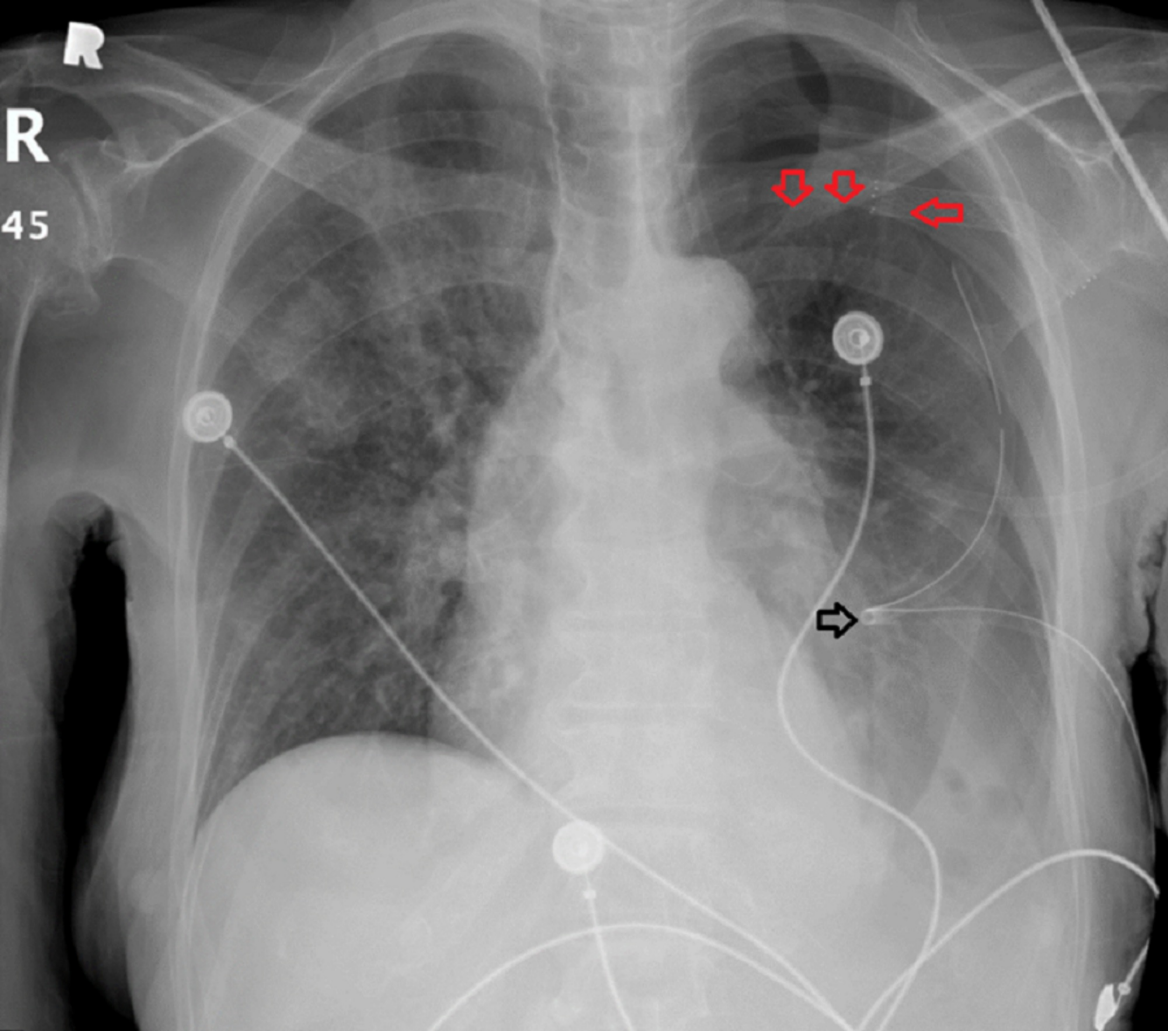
Chest X-ray taken after the first chest tube placement Placement of first chest tube (black arrow), and incomplete resolution of pneumothorax (red arrows).
